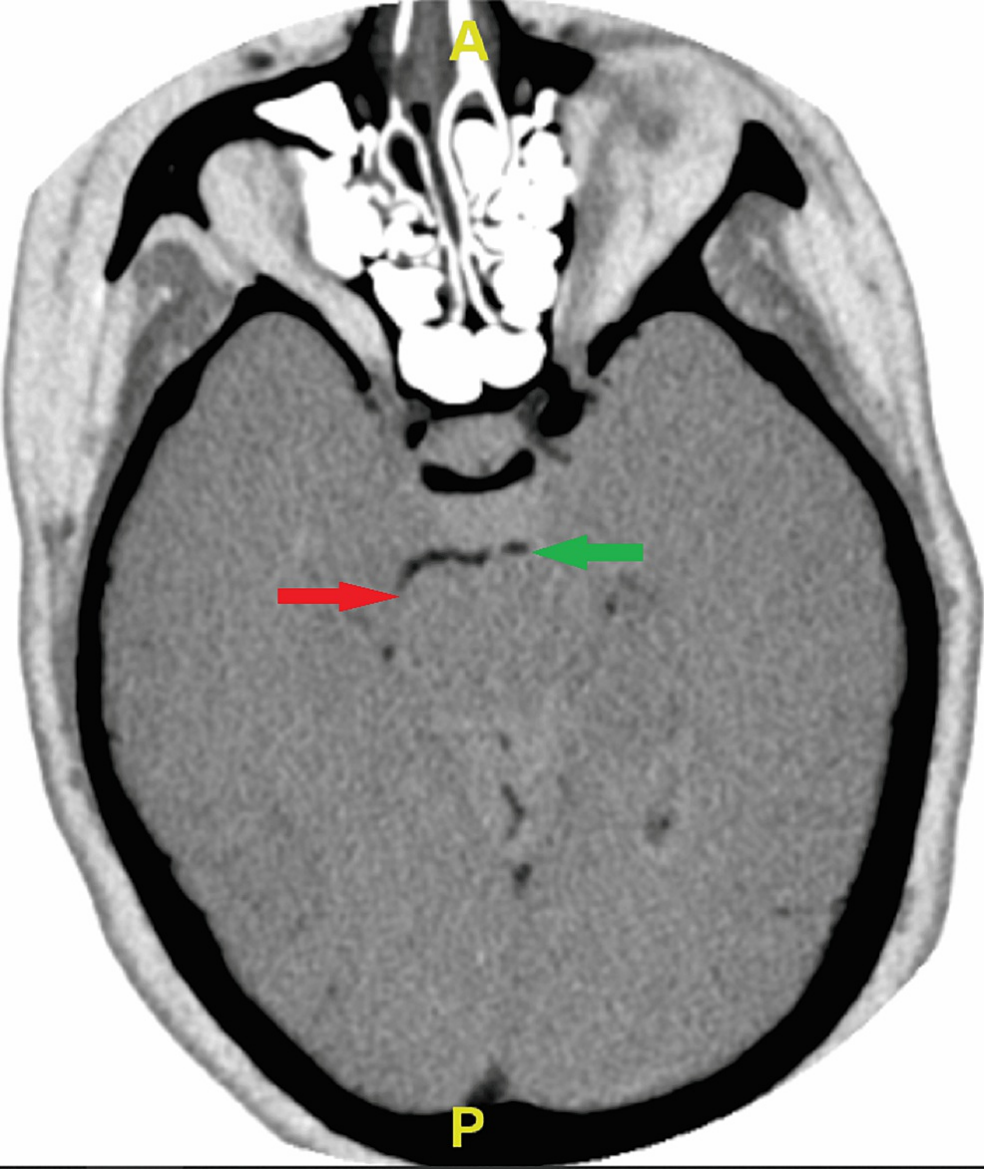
Inverted image of the CTA of the head and neck showing occlusion of the posterior cerebral arteries Axial view of the CTA of the head and neck. The areas of arterial occlusion are indicated as follows: red arrow indicates occlusion of the right posterior cerebral artery at the P3 level, while the green arrow indicates occlusion of the left posterior cerebral artery at the P2 level. Areas in black proximal to the occlusions indicate areas of normal blood flow. CTA: computed tomography angiography
